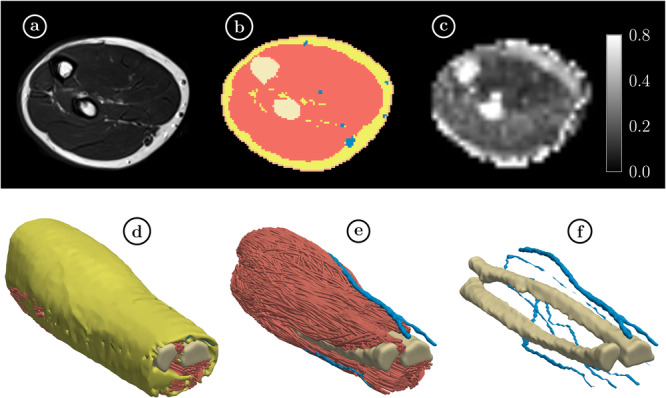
Data from an example forearm model. (a) T2‐weighted MRI; (b) segmented tissues; (c) fractional anisotropy in the axial plane; surface models of (d) fat, (e) muscle fibres, and (f) bone and blood.
